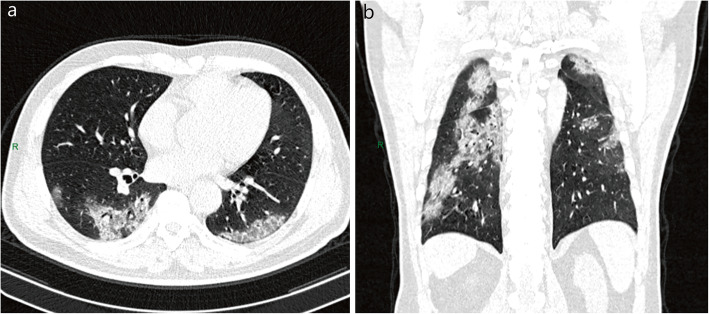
Chest CT of severe patient. A 53-year-old male patient with cough and fever for 6 days. Patchy ground-glass opacities were seen in both lungs, the central density increased, and the lesions were mainly distributed under the pleura. Axial (a) and coronal (b) sections in lung window. R: right
